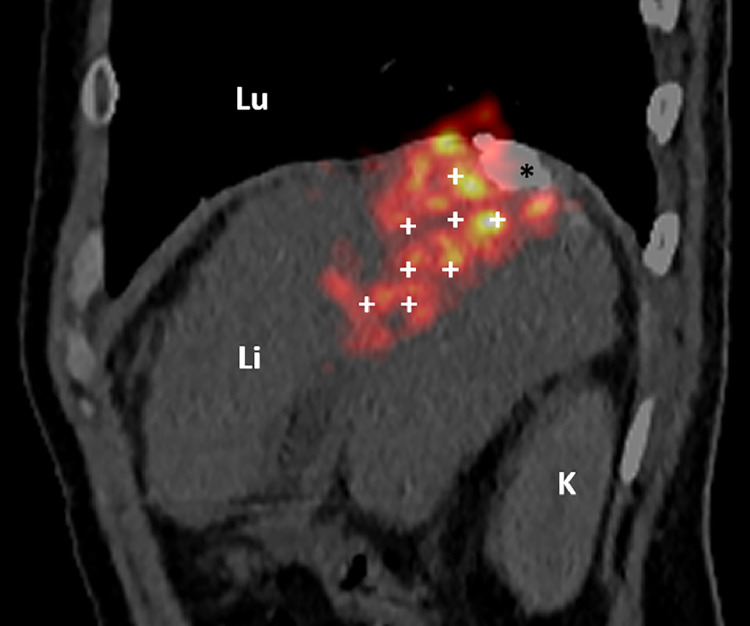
^90^Y PET-CT image showing the treatment with yttrium 90 of the main part of the tumor (white crosses) and the hyperattenuation of the Lipiodol emulsion of the outer part of the lesion (asterisk). Li: liver, Lu: lung, K: kidney.
